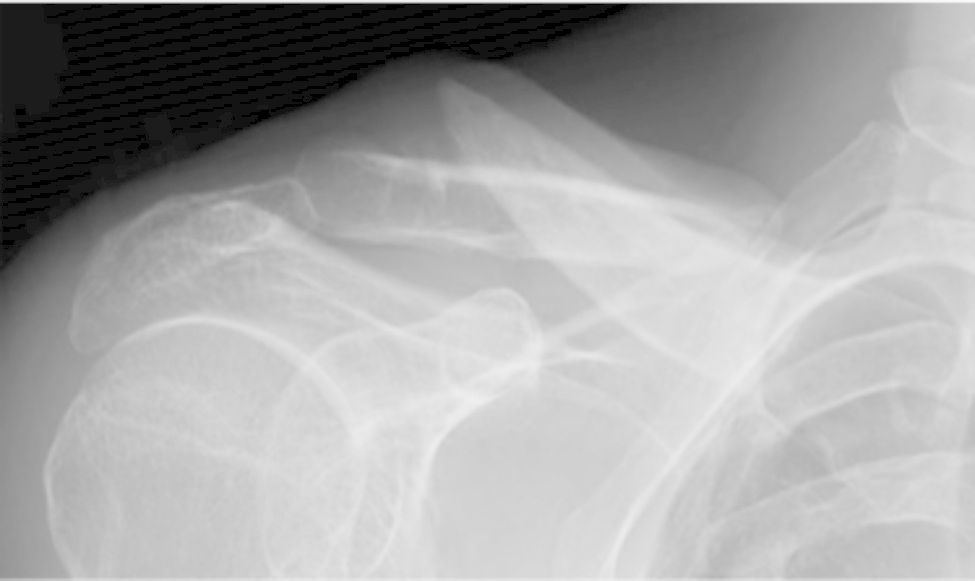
Preoperative radiographs showing atrophic nonunion with a large overlap of the fracture ends
